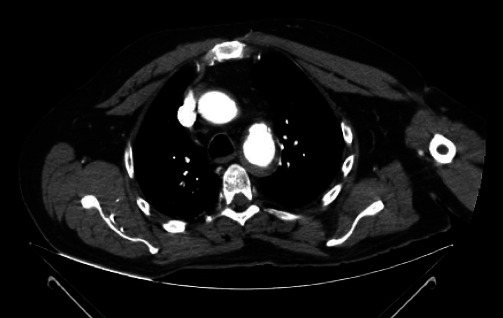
Axial computed tomography scan of the chest performed on a 65-year-old male patient after a frontal collision accident. Aortic dissection is observed at the level of the arch and proximal third of the descending aorta.
